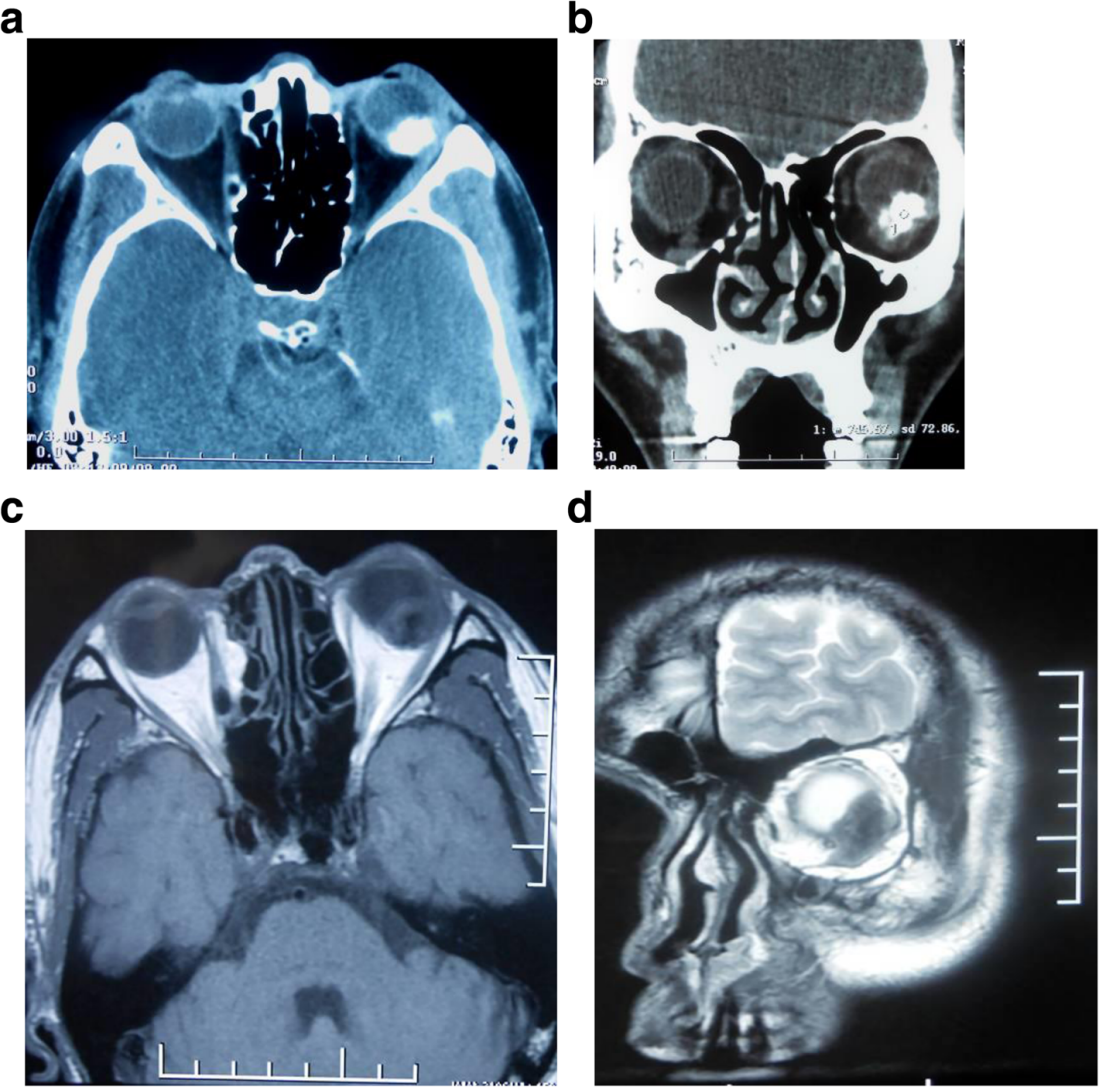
a, The transverse computed tomography revealed an atypical, well-circumscribed homogeneous calcified mass attached the posterior globe in the left orbit. b, The coronal computed tomography showed a calcified mass neither origined from the globe nor the bony orbital wall. c, The transverse T1WI section showed the heterogeneous lesion .The lesion was approximately 17.7 × 14.1 × 24.2 mm in the orbit. d, The coronal T2WI section showed the heterogeneous lesion presents hypointense in the left orbit
